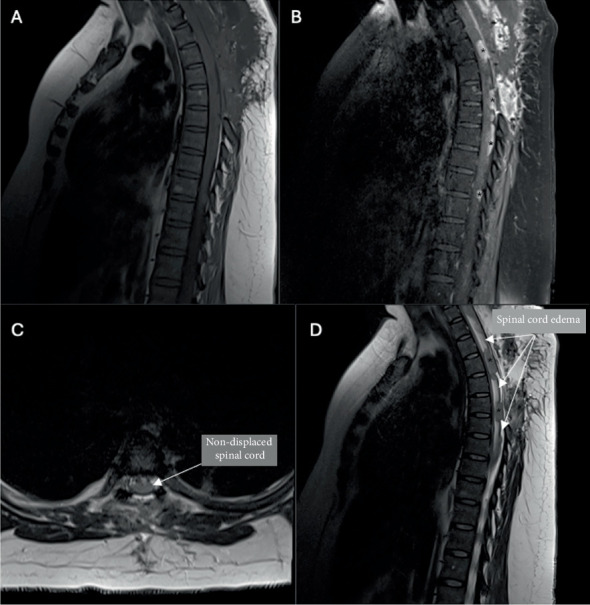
Postoperative images. Postoperative T1 sagittal MRI (A) without contrast and (B) with contrast demonstrating residual tumor with significantly less volume (⁣^∗^) and spinal cord compression. Postoperative (C) T2 axial MRI without displaced cord and (D) T2 sagittal MRI with continued spinal cord edema.
